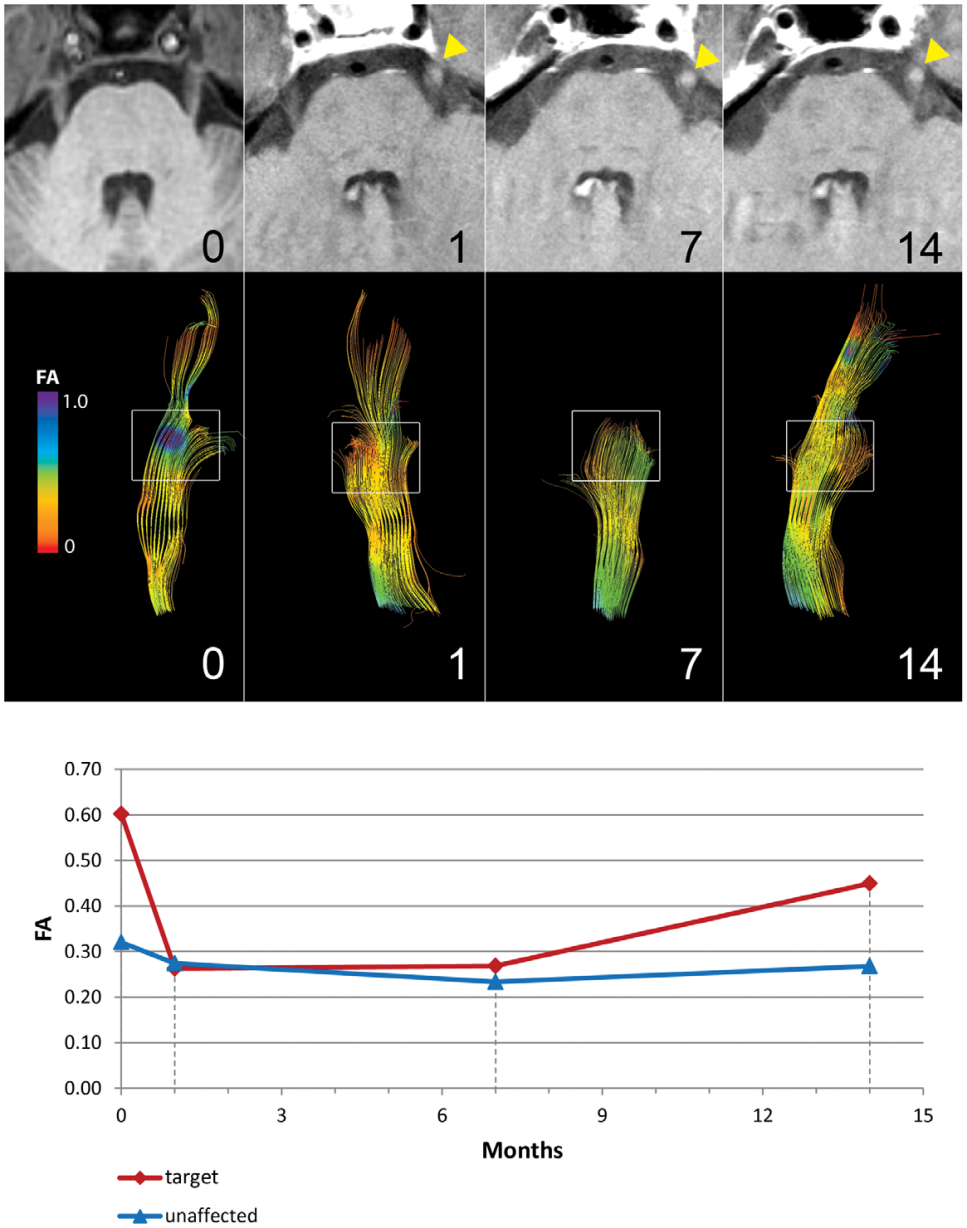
Changes in FA after GKRS treatment are dynamic. Sequential images for subject S1 are shown at 0, 1, 7 and 14 months after Gamma Knife radiosurgery (GKRS) treatment. Top panel depicts serial MR images, showing similar gadolinium enhancement in the midcisternal portion of the nerve after treatment with time (yellow triangles). Middle panel shows reconstructed trigeminal nerve tracts for the same time points. At one month, marked FA decrease is seen in the target area (high FA pre-treatment, blue now appearing as low FA, orange) and tract-pruning due to fall-off of FA value. At 14 months the FA values trends towards baseline, with a longer reconstructed trigeminal segment. The area of low FA has also resolved. Lower panel shows a graph of the scalar values of FA with time. At 14 months, subject S1 has experienced full recurrence of her pain.
